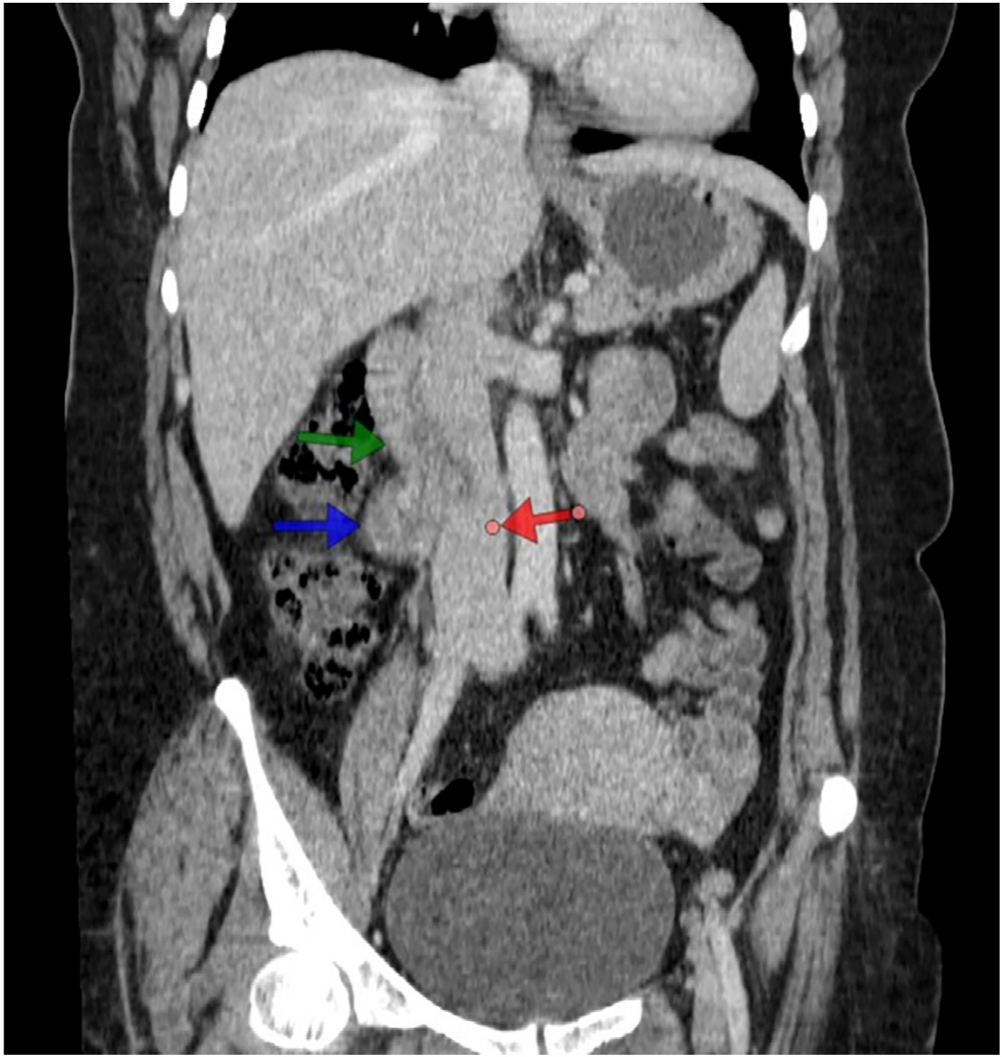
Contrast-enhanced CT of the abdomen, coronal section, shows a retroperitoneal enhanced mass “blue arrow”, the second part of the duodenum superior to the mass and in contact with it “green arrow”, and the IVC medial to the mass “red arrow”.
